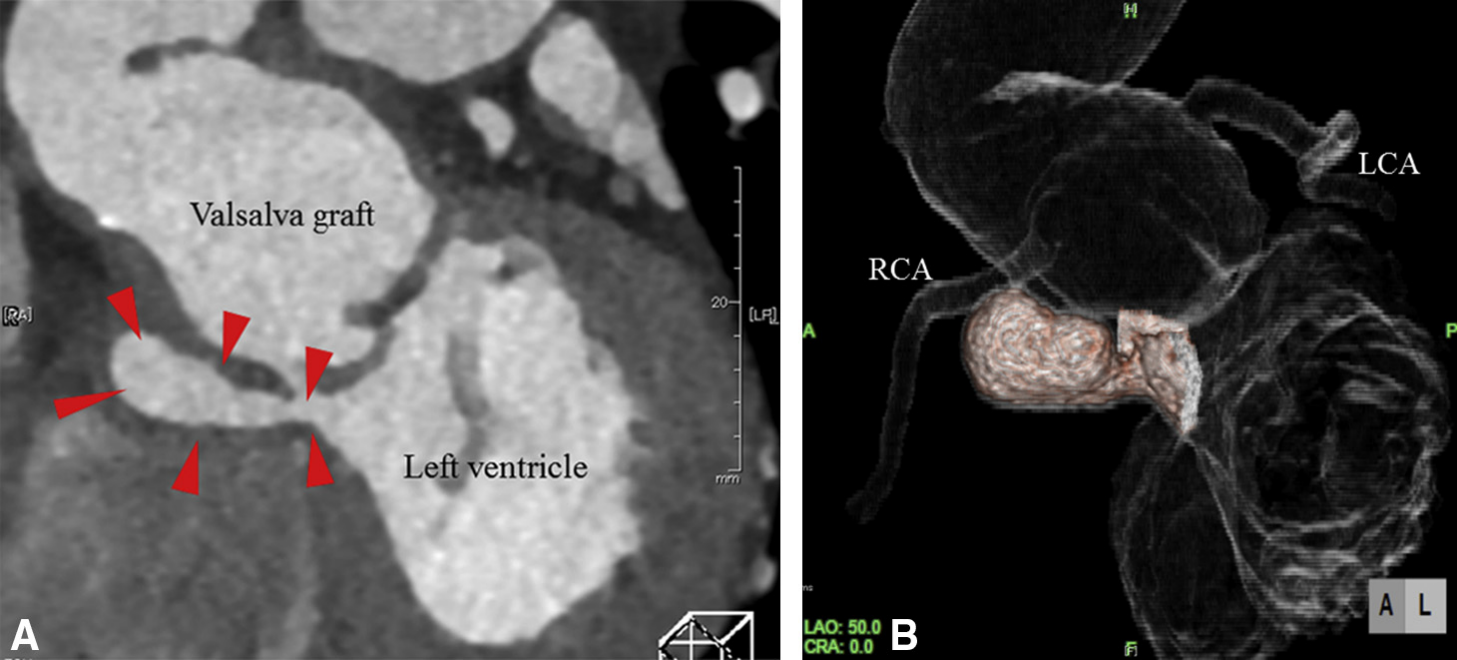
A, Preoperative contrast-enhanced computed tomography of the aortic root shows pseudoaneurysm formation (*red arrowhead*) from the left ventricle outflow tract. B, Preoperative 3-dimensional computed tomography of the aortic root. *RCA*, Right coronary artery; *LCA*, left coronary artery.
